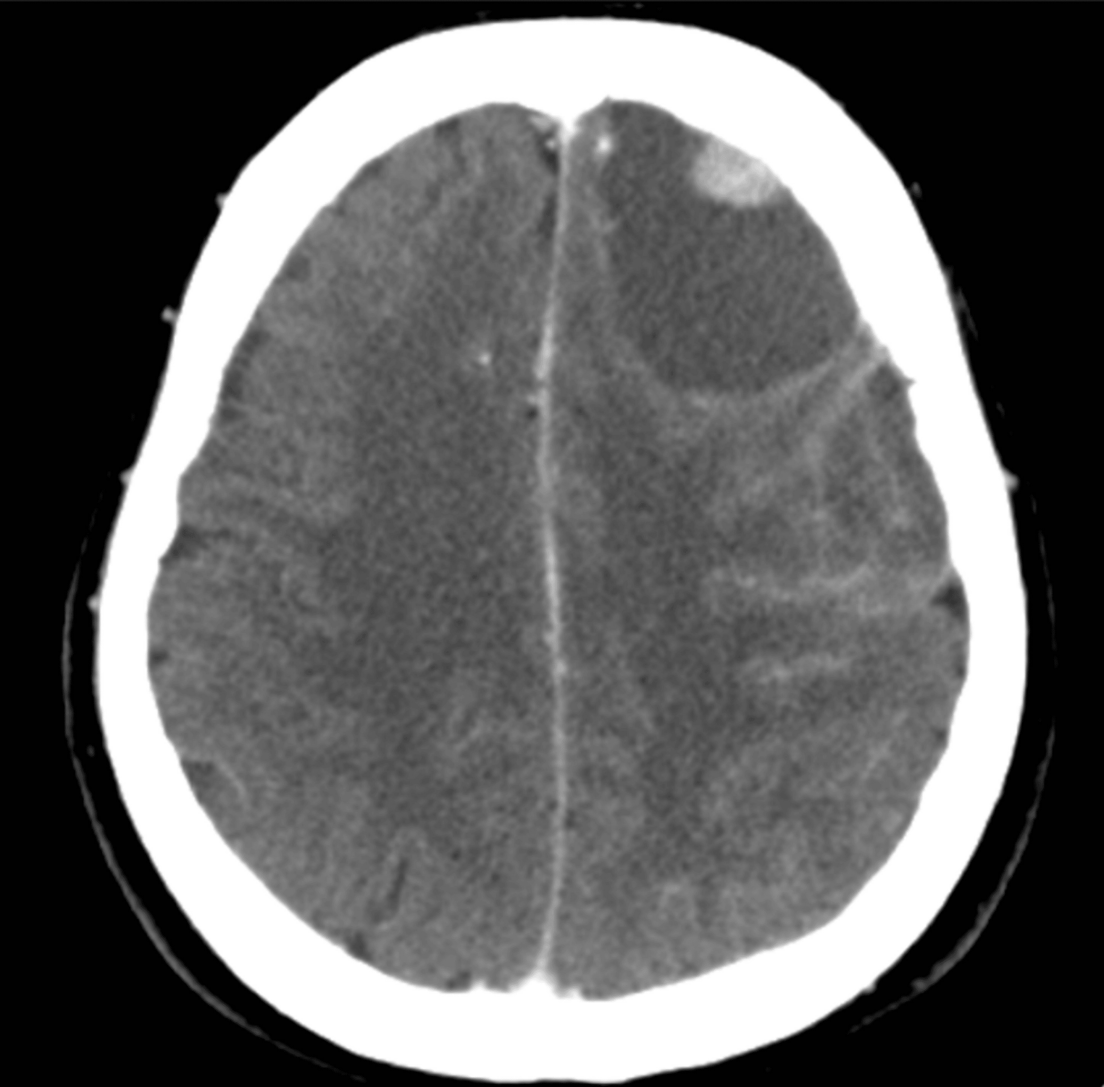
Brain CT without contrast of case 2 A heterogeneous, globular lesion in the left high frontal region. The differential diagnosis included cystic meningioma, hemangioblastoma and metastatic tumor.
